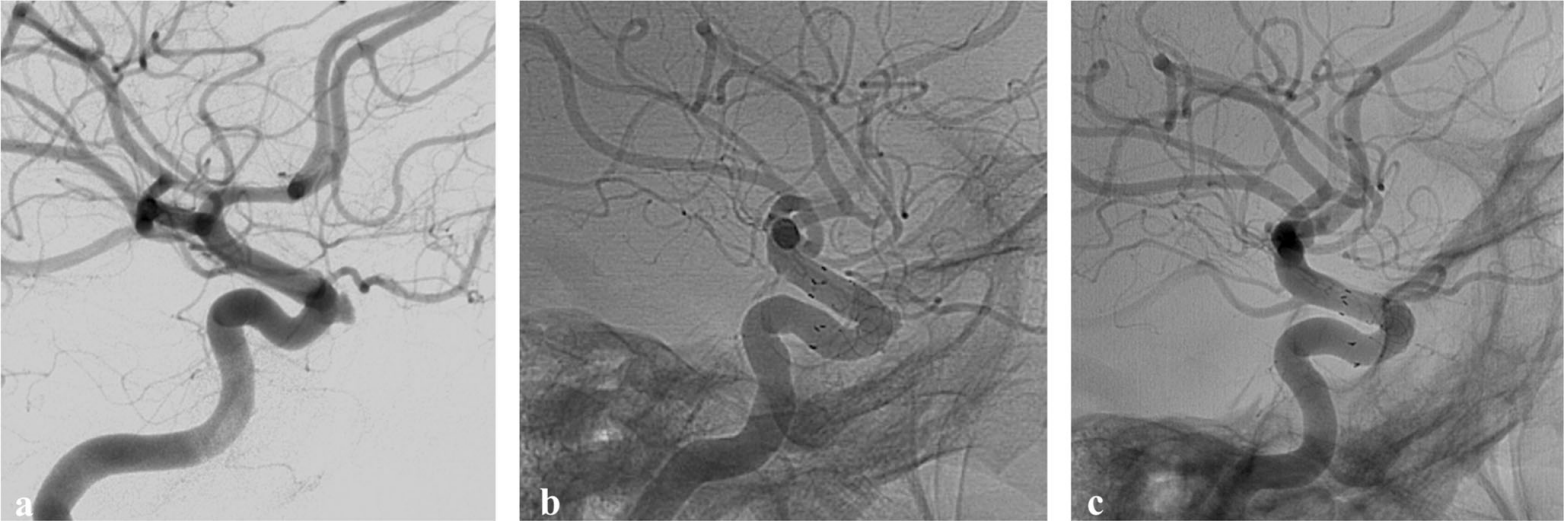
Case #3 is a 48-year-old woman admitted to our institution for sudden epistaxis. She was affected by recurrent chronic rhinosinusitis with nasal polyps treated at another center with radical spheno-ethmoidectomy with maxillary and frontal sinusotomies about 1 month before. A diagnostic CTA and DSA documented a PSA of the anterior profile of the left carotid siphon. An FDS (FRED, 4 × 12/18 mm) was placed. a Pre-procedural DSA showing PSA of the anterior genu right cavernous ICA segment. b Intra-procedural DSA showing FDS correct placement. c DSA at 3 months, demonstrating the regular cavernous ICA profile, without evidence of PSA recurrence
